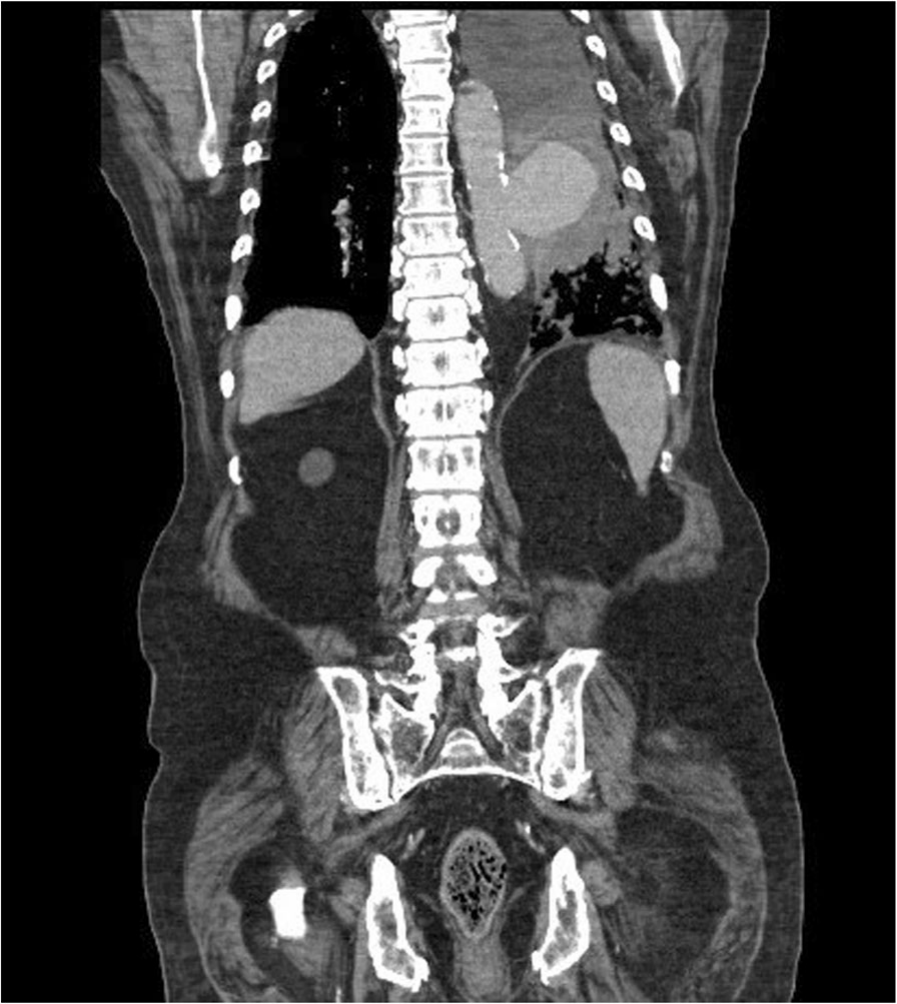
Computed tomography scans with intravenous contrast media show a thoracic aortic aneurysm with hemothorax
